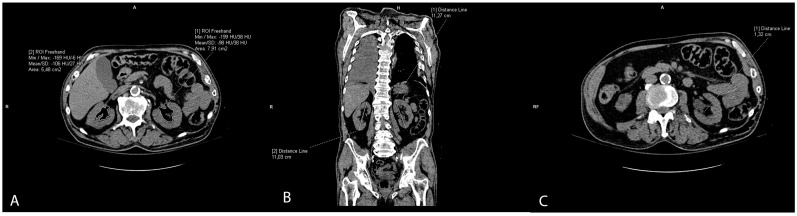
Representative CT scans showing renal parameter volume measurements: renal sinus fat area (A), length of the kidney (B), and renal parenchymal thickness (C).
